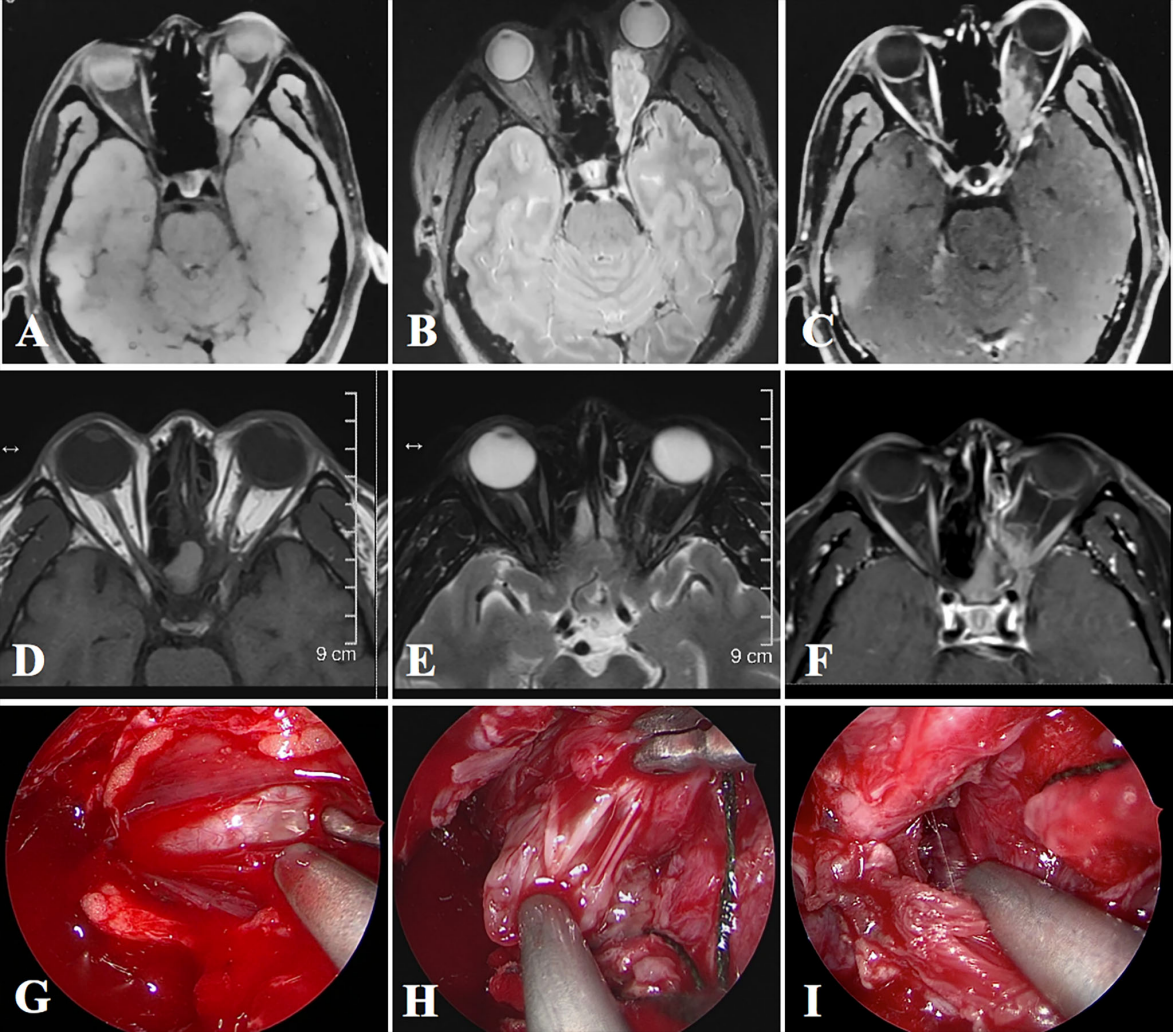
Images of Case 2. (A–C) Preoperative MRI shows an intraconal lesion in the left orbit, with both medial and lateral extension. (D–F) Six-month follow-up MRI shows no recurrence of the tumor. (G) Exposure of the intraconal tumor. (H) The carrier nerve of the tumor. (I) Inspection after total resection of the tumor.
